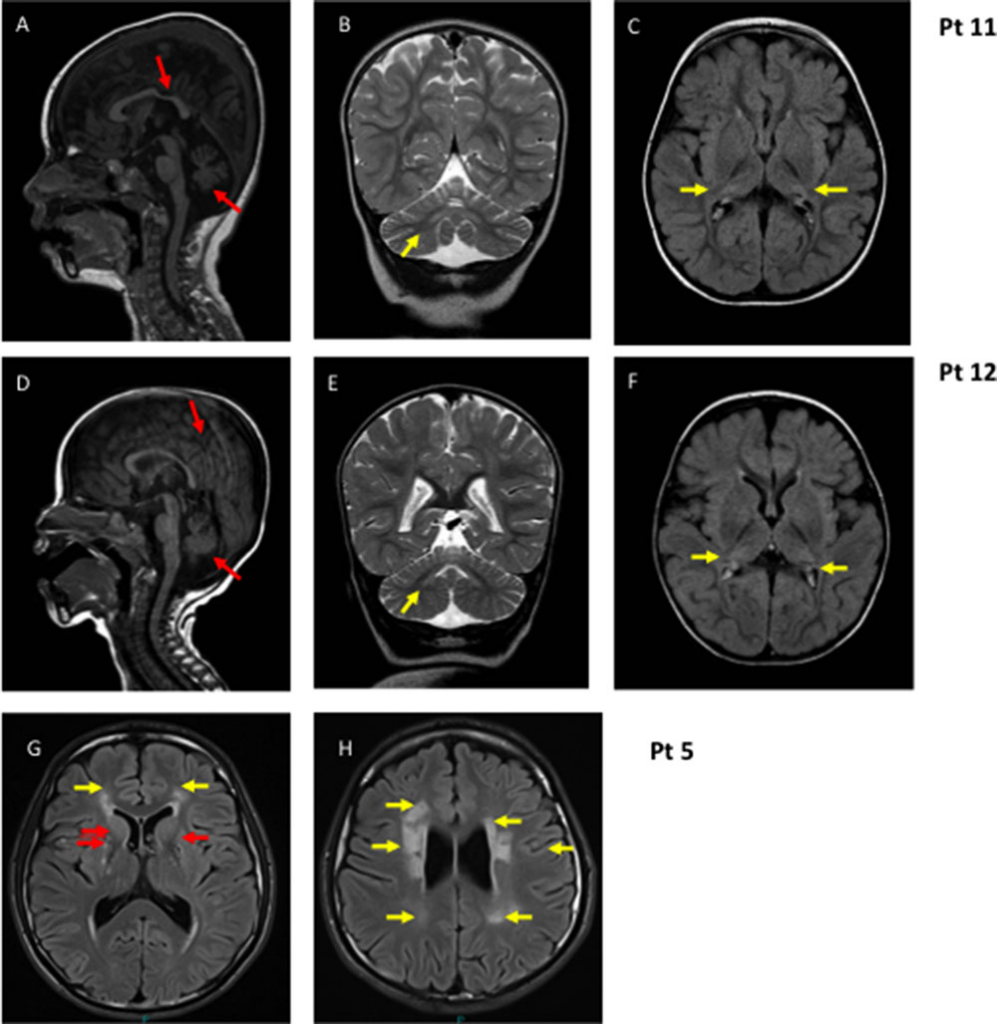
MRI: Sagittal T1 (A and D), coronal T2 (B and E), and axial flair (C and F) images of P11, age 2 years and 4 months, and P12, age 13 months, showing cerebellar atrophy, thinning of the posterior corpus callosum (red/dark arrows), signal intensity in dentate nuclei and pathological signal in the lateral parts of thalami that look smaller (yellow/light arrows). G and H: Axial flair images of P5, age 15 years: yellow arrows indicate diffuse symmetrical periventricular white matter abnormality; red arrows indicate basal ganglia calcification
